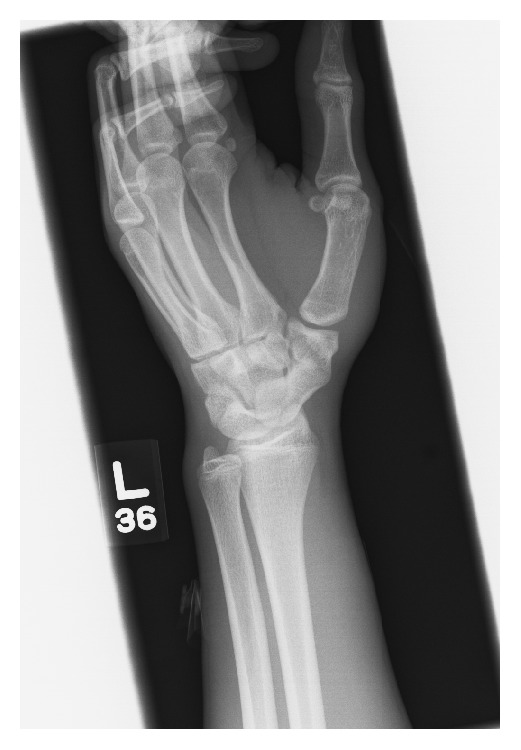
Oblique radiograph of the wrist demonstrates isolated scaphotrapezial coalition.
